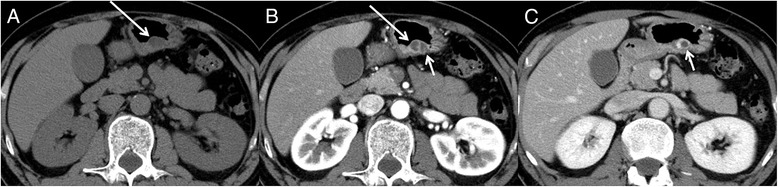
Dynamic enhanced computed tomography (CT) (a, non-enhanced CT; b, arterial phase; c, delayed phase) revealed a gastric subepithelial tumor at the posterior wall of the gastric angle. Long arrow indicates the cyst shrank, and short arrow indicate the cyst enlarged on the follow-up CT
